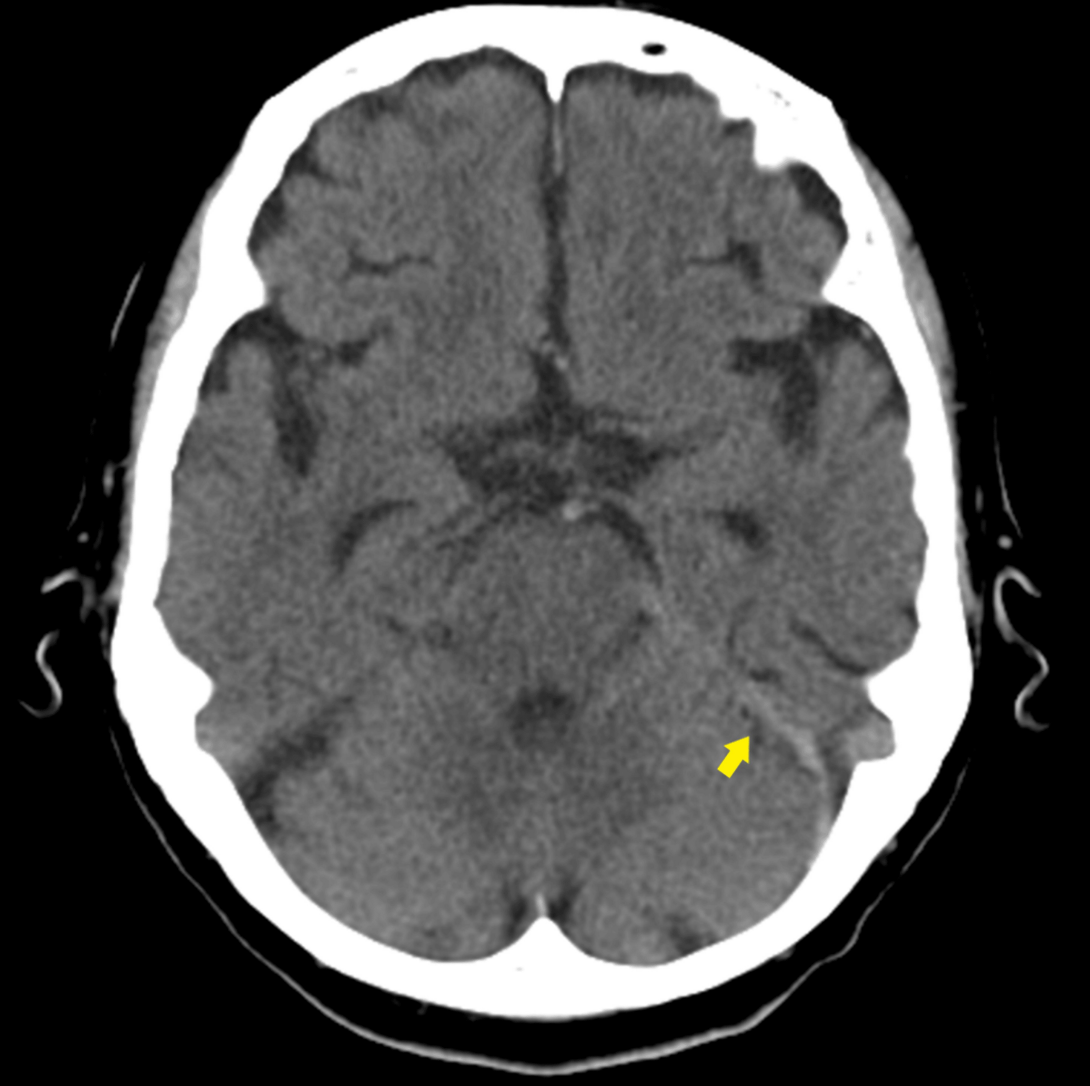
Brain CT A brain CT scan revealed a 4-mm-thick subdural hematoma along the left tentorium (yellow arrow), without midline shift or skull fracture.
